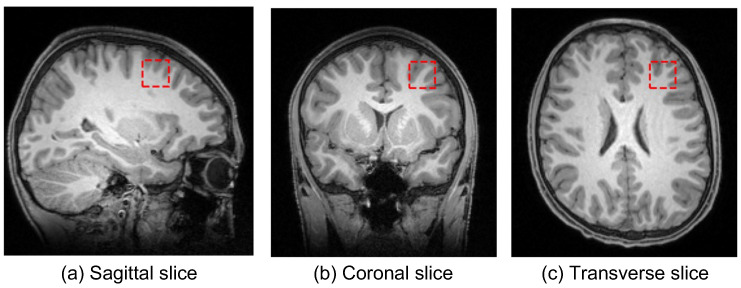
The T1-weighted MRI and the left MFG region. Three subplots are (a) a sagittal slice from left to right, (b) a coronal slice from top to bottom, and (c) a transverse slice from back to front, respectively.
